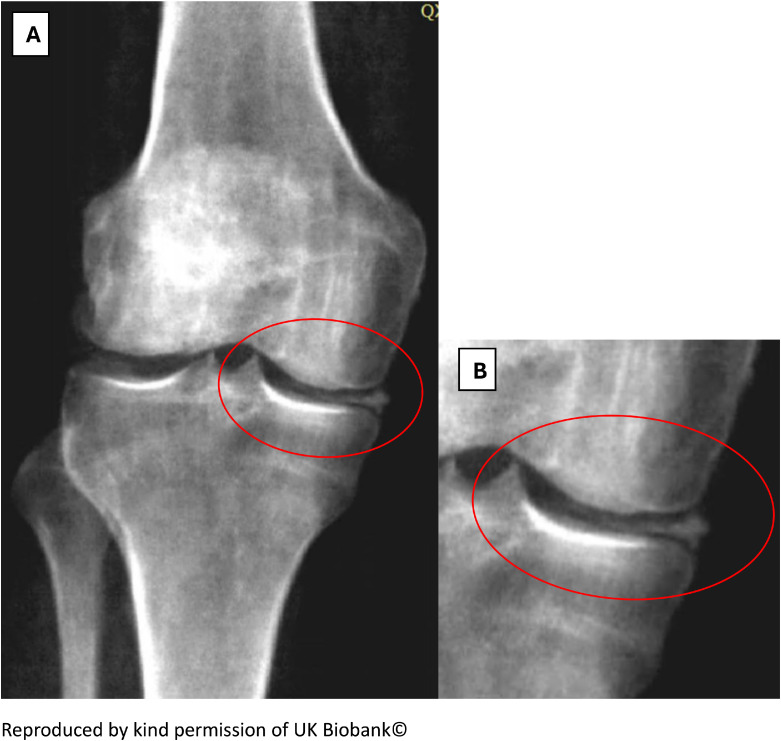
Example of chondrocalcinosis of the knee on an iDXA image in UK Biobank (A) with an enlarged picture (B) to highlight the presence of chondrocalcinosis in the medial tibiofemoral space. Reproduced by kind permission of UK Biob ank©. iDXA, intelligent dual-energy X-ray absorptiometer.
